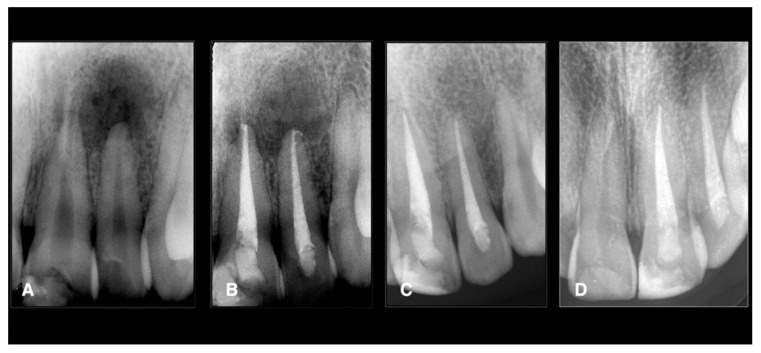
Endodontic treatment of a second maxillary left incisor. The tooth was necrotically associated with periapical lesions. The treatments were done using conservative shaping and the 3D cleaning procedure, as mentioned in the Materials and Methods. (A) Preoperative X-ray, (B) postoperative X-ray, (C) 1-year follow-up, (D) 2-year follow-up showing healing.
